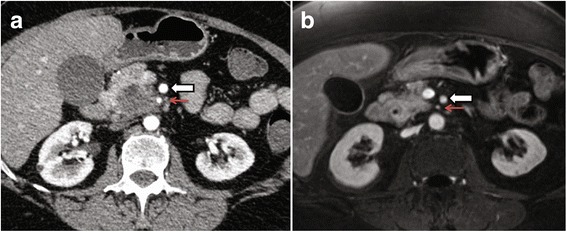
Abdominal CT scans. a The mass is separate from the celiac axis an there is loss of a fat plane between the mass and SMA (*white arrow)* with less than 90° involvement of its circumference. The first arterial jejunal branch (*red arrow*) is also encased with tumor. There is also mild narrowing of the medial aspect of the superior mesenteric vein where the tumor abuts around 90° of its circumference. The pancreatic duct is dilated, measuring up to 6 mm. b Following 9 cycles of FOLFIRINOX, the follow up MRI reveals that the head of the pancreas has indistinct margins, but has clearly diminished in size. The measured size is 2.7 × 2.3 mm (prior: 3.9 × 3.2 mm). Pancreatic ductal dilatation has subsided, indicating response to treatment
